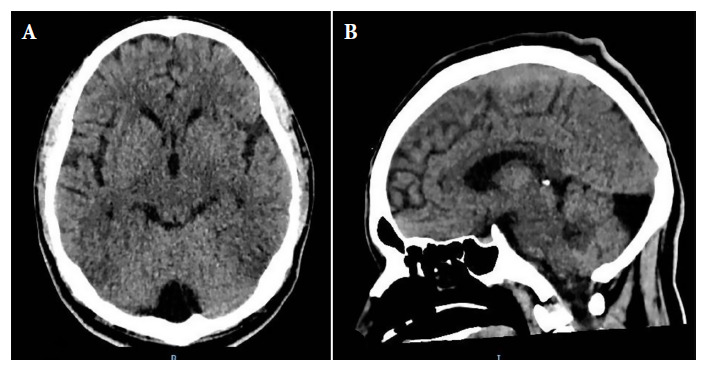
Non-contrast brain CT scan. Axial (A) and sagittal (B) views show a rounded, hypodense image with liquid density located in the midline of the posterior fossa, suggesting an arachnoid cyst. The rest of the brain parenchyma appears normal.
